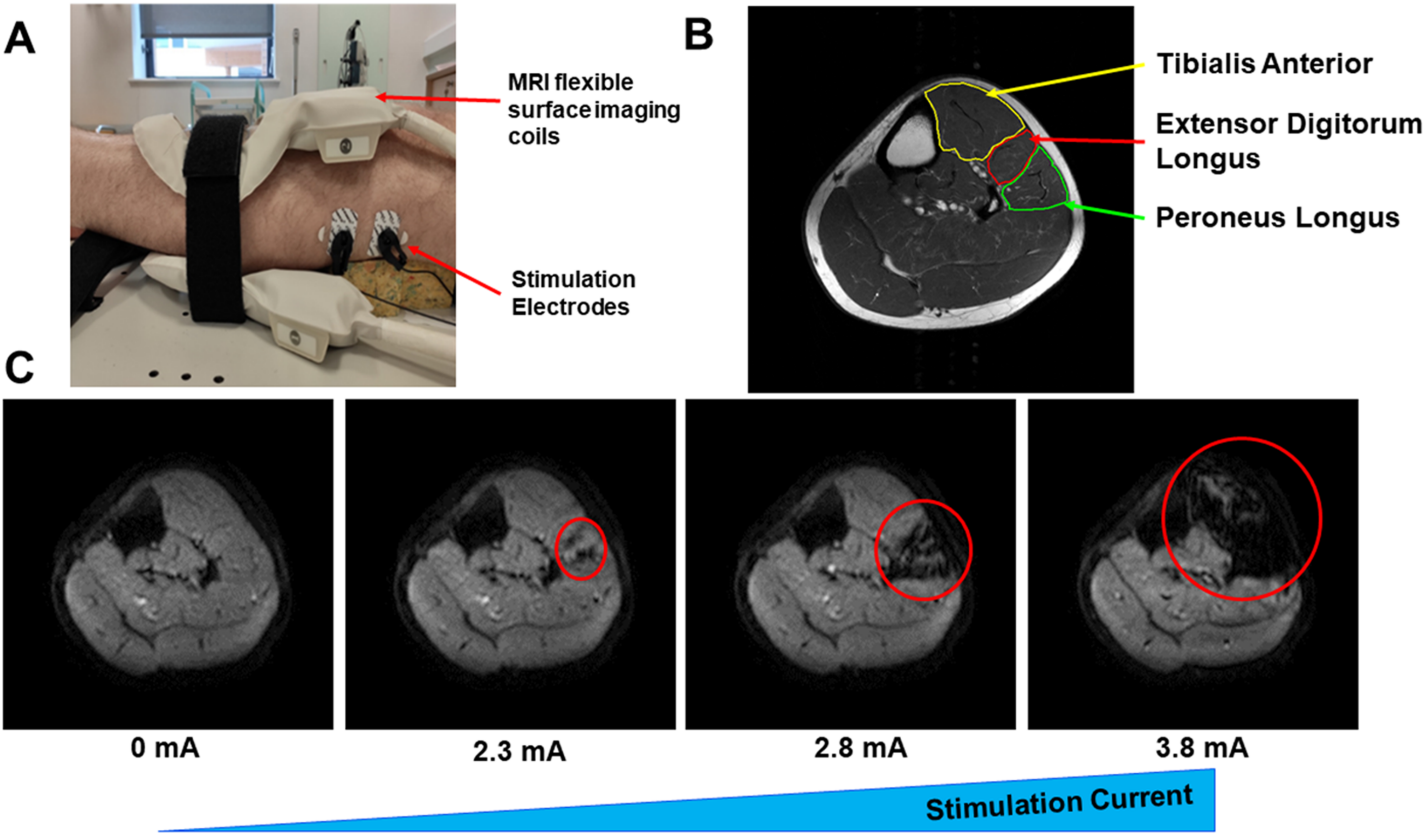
An example of the motor unit magnetic resonance imaging setup and resulting images. (*A*) Example experimental setup for stimulation of the fibular nerve which innervates muscles of the anterior compartment. (*B*) Axial T1w magnetic resonance imaging of the lower leg muscles, muscles of the anterior compartment are indicated (yellow—tibialis anterior, red—extensor digitorum longus, and green—peroneus longus). (*C*) Four axial diffusion‐weighted images of the lower leg muscles. Left image is with no stimulus applied, and the volunteer is at rest. As the stimulus current is increased, motor unit activity indicated by the red circles can be observed beginning in the *extensor digitorum longus* at 2.3 mA; eventually, the whole anterior compartment has been activated at 3.8 mA.
